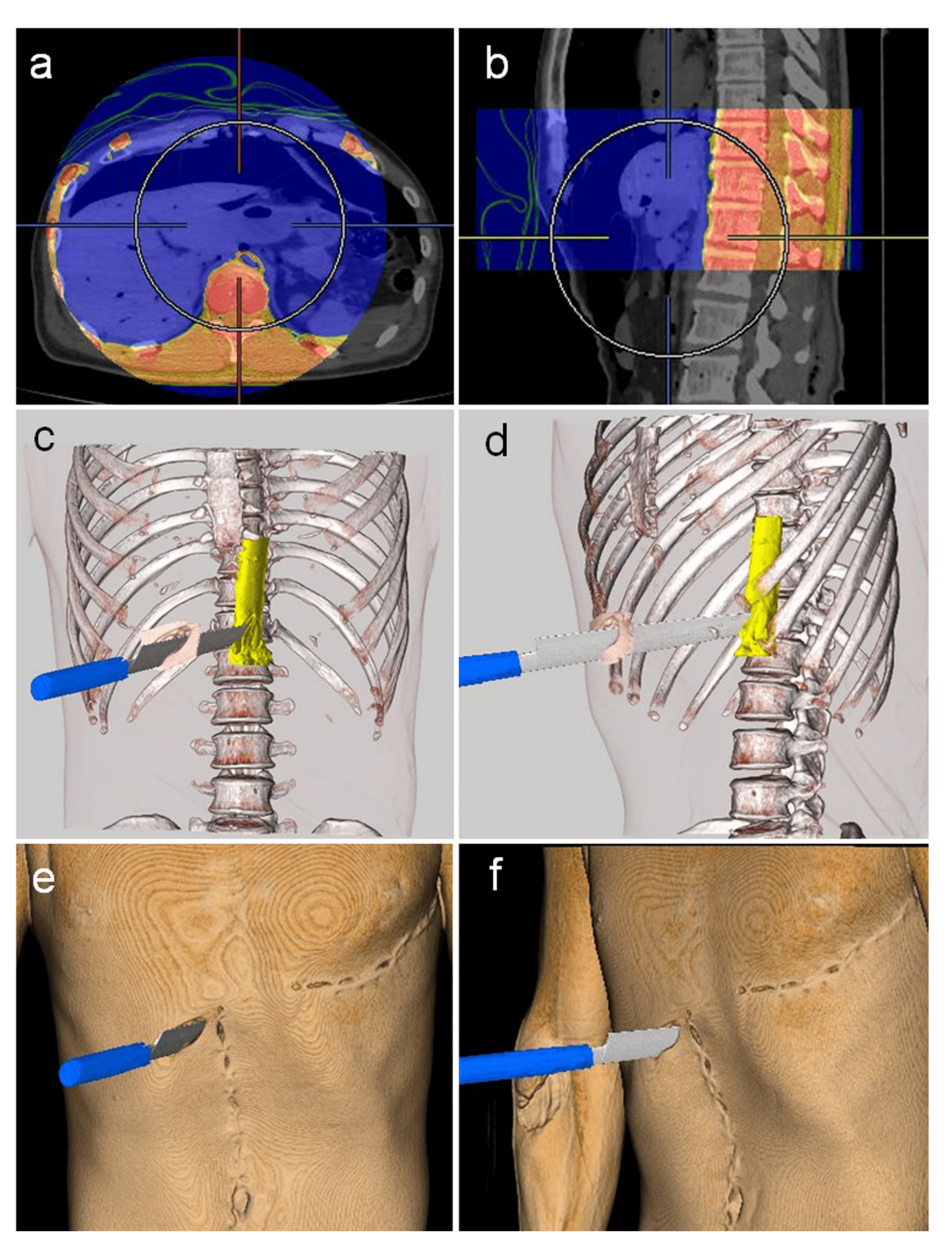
Superimposed images obtained using pre-autopsy, intra-autopsy, and knife CT data. a, b. Alignment of pre-autopsy and intra-autopsy CT data. a. Axial 2D image at the level of the 11th thoracic vertebra. b. Axial 2D CT image. Intra-autopsy CT images are displayed using a pseudocolor scale, whereas pre-autopsy CT images are shown in grayscale. c, d, e, f. 3D superimposed images were obtained using pre-autopsy, intra-autopsy, and knife CT data. c, d. Superimposed images were obtained with transparent skin except for the right hypochondriac region. c. Frontal view. d. Left lateral view. e, f. Superimposed images with the entire skin set to brown. e. Frontal view. f. Left lateral view.
